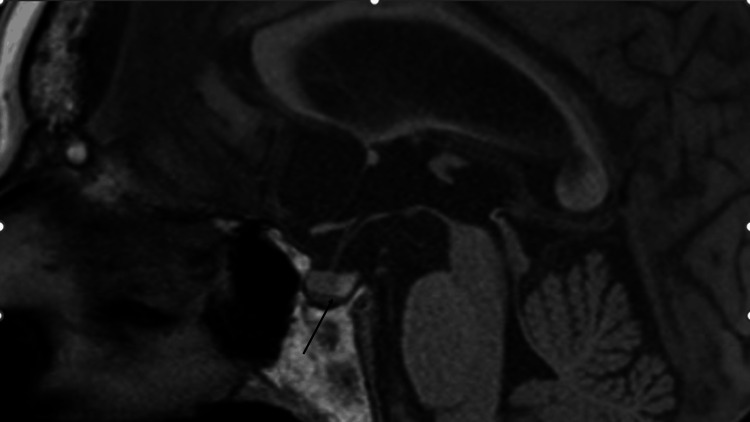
T1-weighted image in pituitary magnetic resonance imaging (MRI). Pituitary MRI shows normal findings. Arrow shows normal findings of the pituitary.
